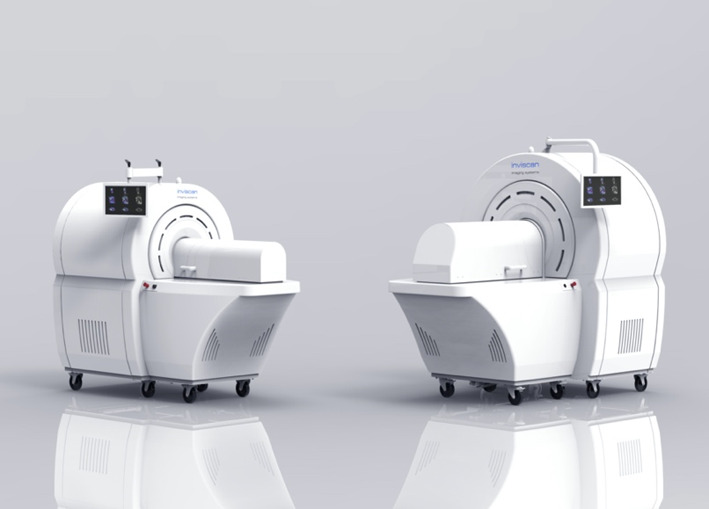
Photographs of the IRIS PET/CT (on the left-end side) and the IRIS XL-220 PET/CT (on the right-end side) systems at the core facility for preclinical imaging of the University of Tours (Département d’Imagerie Préclinique, Plateforme Scientifique et Technique Analyse des Systèmes Biologiques, Université de Tours, Tours, France)
